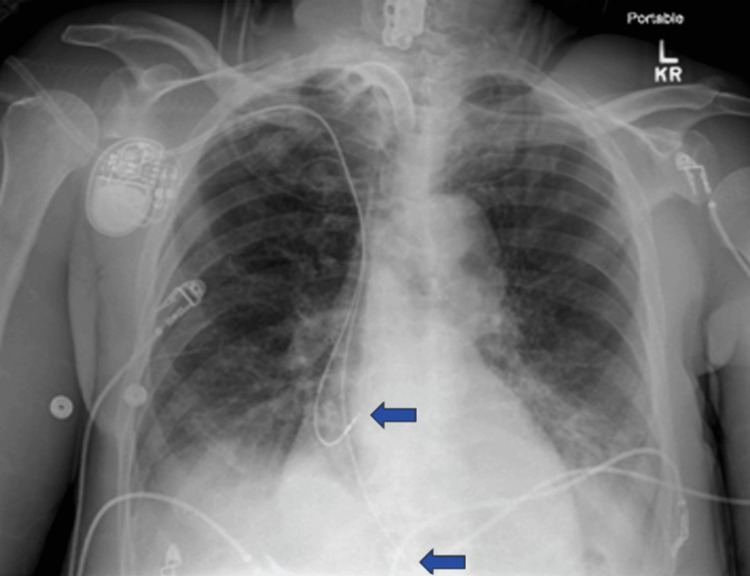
Chest X-ray showing complete resolution of the left-sided whiteout with realignment of the pacemaker leads (blue arrows).
